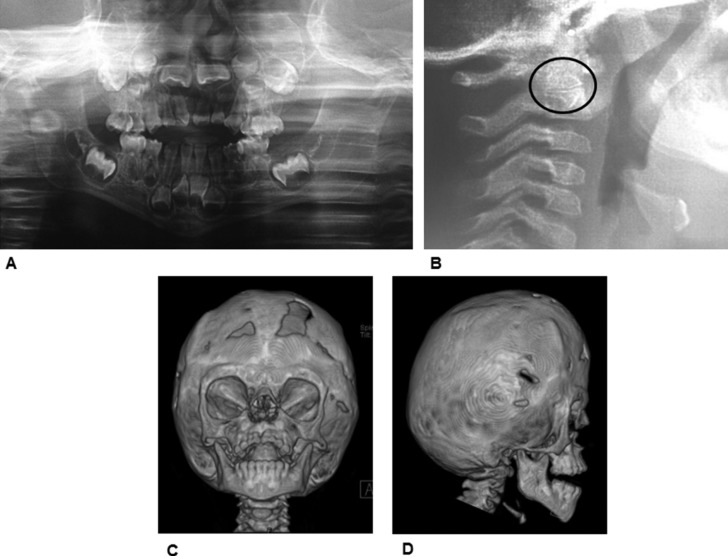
A. Panoramic view. Note the abnormal eruptive process of both permanent upper central incisors. B. Note the partial fusion between C2 and C3 body vertebrae, in their distal portions. Skull three-Dimensional (3D) Computed Tomography (CT) views. C. Front D. Right. Hypoplastic midface and skeletal anterior open bite are evident.
